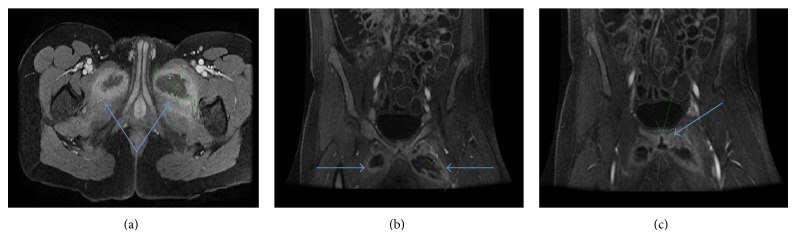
Preoperative MRI showing ((a), (b)) large fluid collection within the medial compartment of the thigh bilaterally, extending along the adductor brevis and adductor longus muscles. (c) The abscess on the left side extending more superiorly to the midline with fluid in the symphysis pubis joint suggestive of an effusion and symphysis.
